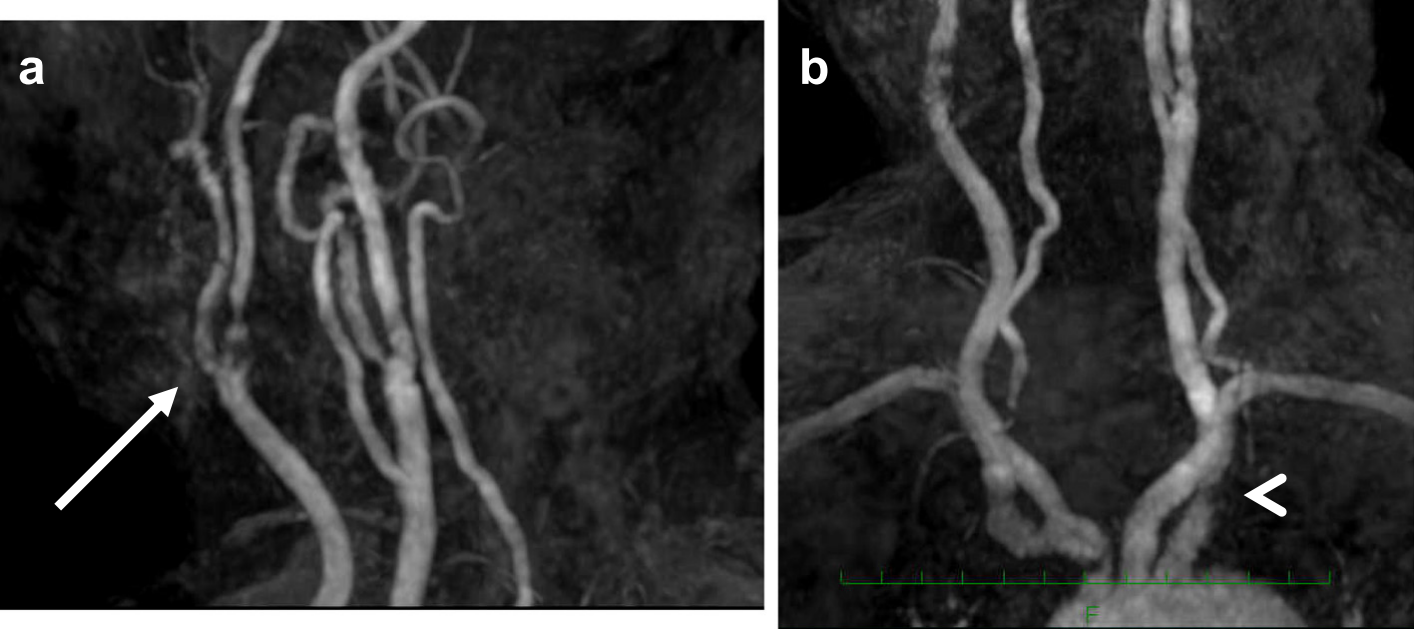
Magnetic resonance imaging showing stenosis of the right internal carotid artery (arrow) (a) and the left subclavian artery (arrowhead) (b)
